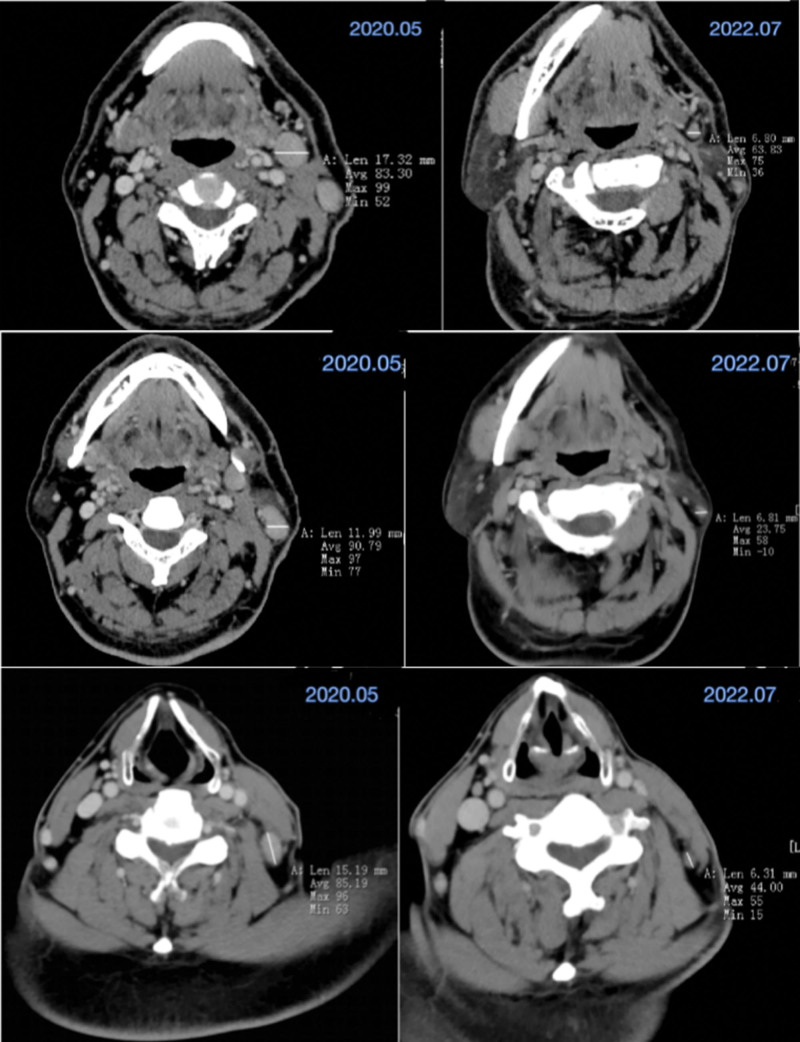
CT images show changes in lymph node size at different levels before and after treatment.
